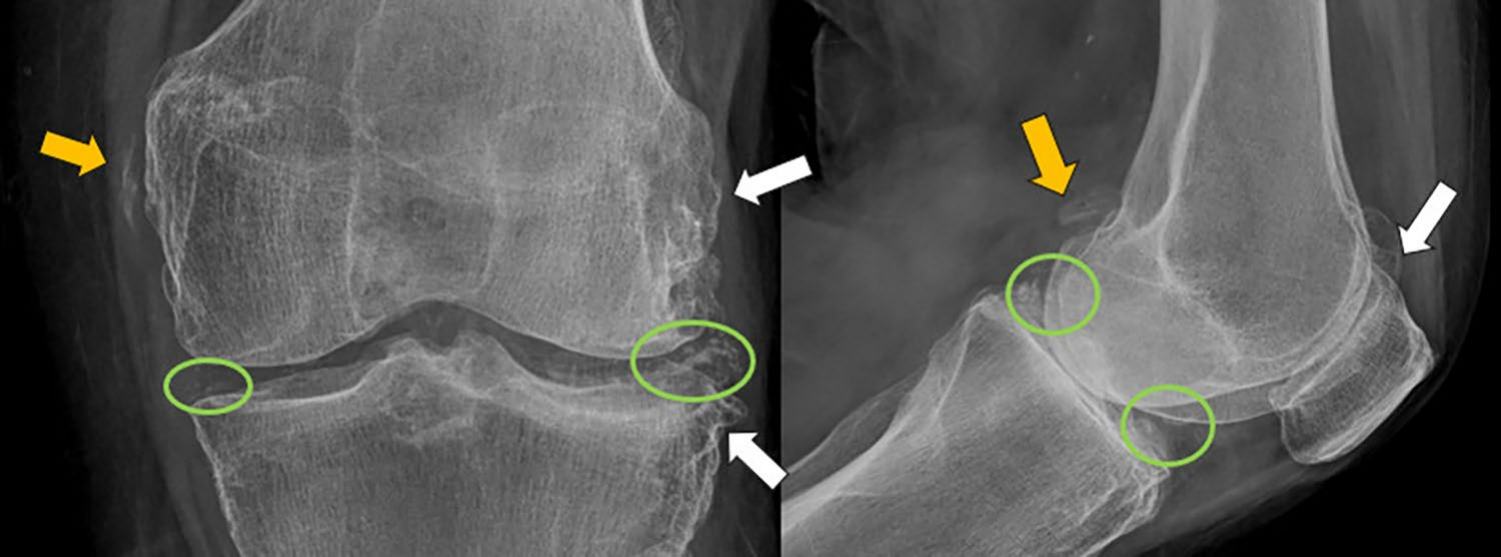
AP and L views of the right knee in a 60-year-old woman with left-sided gonalgia associated with increased joint volume and documented signs of chondrocalcinosus and pyrophosphate arthropathy. Radiographic ABCDE’S approach: A: Adequate alignment B: Marginal osteophytes of the outer contours of the femoral condyle and lateral tibial plateau (white arrows). C: Radio-opaque punctate foci of lesser density than the bony cortex, located 1-2 mm from the bony cortex, located in the topography of the medial and lateral meniscus (green circles). D: Decreased femorotibial joint space, predominantly left. E: No erosions are observed S: Radio opaque cottony foci compatible with calcification of the fascia lata tendon and gastrocnemius muscle (orange arrows)
